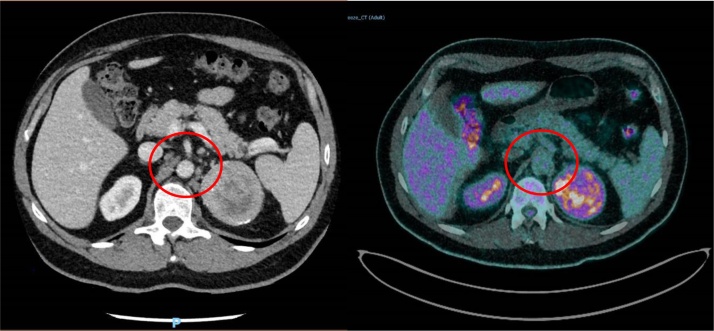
^18^FDG-PET-CT combined with contrast CT-scan showing para-aortic and epigastric not hypermetabolic enlarged lymph nodes.
